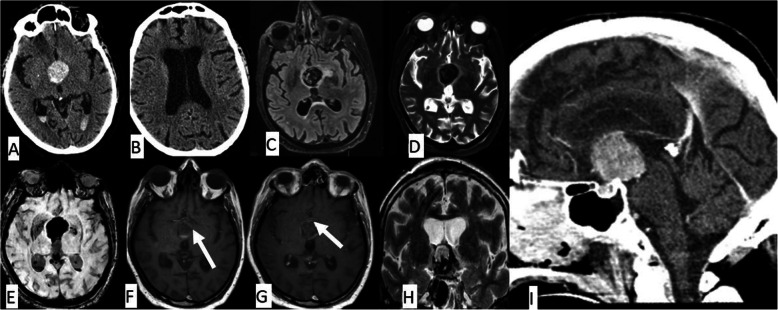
Axial non-contrast CT shows a well-defined hyperdense lesion in the suprasellar cistern associated with intraventricular hemorrhage and acute hydrocephalus (a, b). The lesion is seen as low signal on FLAIR (c) and T2 (d) with a signal drop on SWI (e). The lesion shows laminar morphology on T1 (f) and post-contrast T1 (g) but without enhancement. On the coronal T2 (h), the lesion appears as an exophytic mass from the hypothalamus with peripheral parenchymal edema. On the sagittal post-contrast CT (i), there is a large hyper-dense suprasellar mass with mass effect (compression) over the third ventricle
